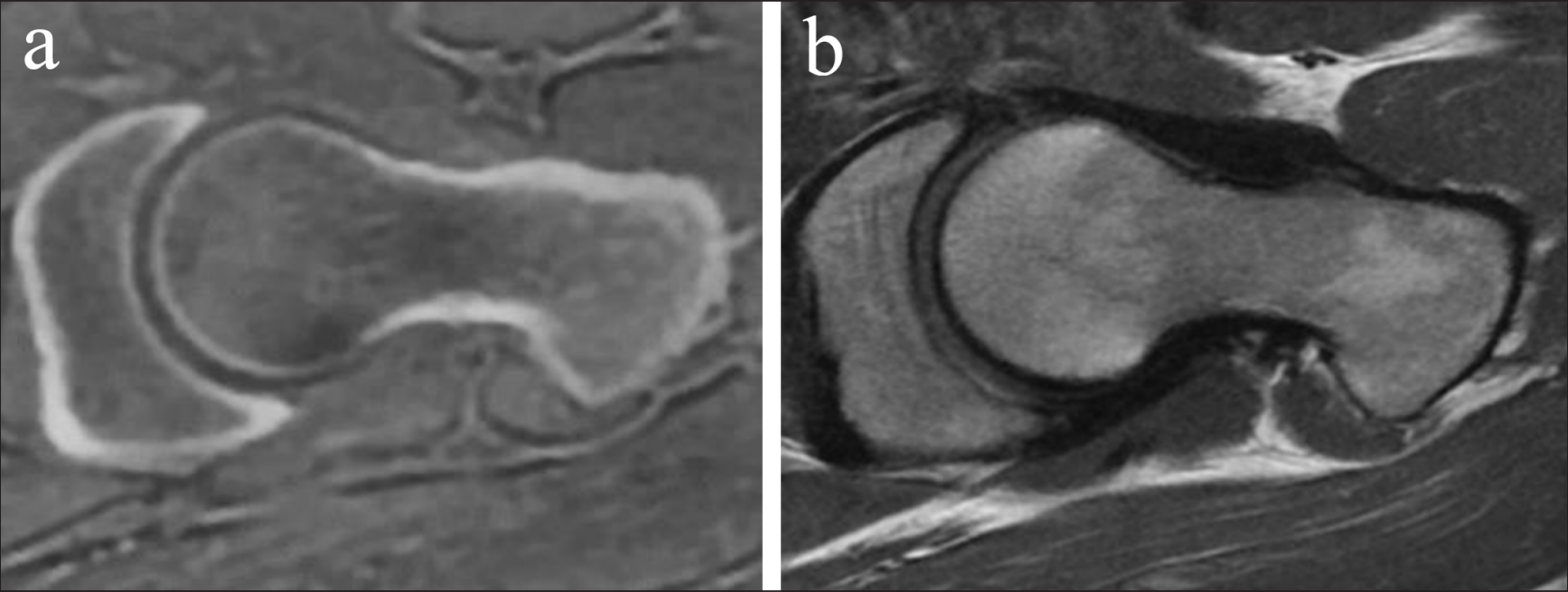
Zero echo time (ZTE) versus proton density weighted images. Swiss axial views of the femur are shown in ZTE MRI (a) and proton density weighted MRI (b). The hypointensity at the cortical margins renders the cortices difficult to distinguish from the joint capsule or the chondrolabral junction. Courtesy of Ryan Breighner, Weill Cornell Medical College Of Cornell University, New York [25].
